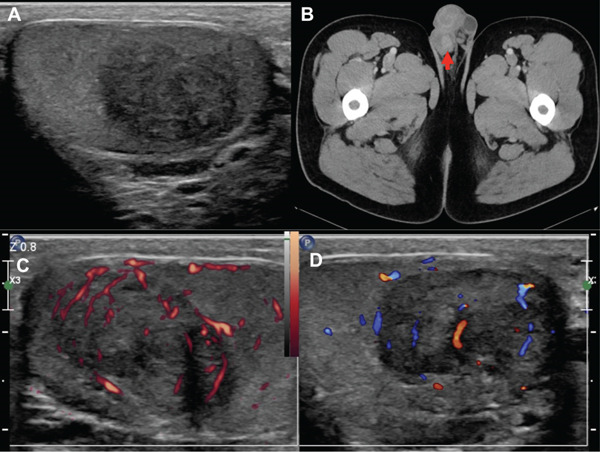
(A) Ultrasound of the right testis demonstrates a heterogeneous hypoechoic 1.3 × 2 × 2 cm lesion along the upper to interpolar region. (B) Non‐contrast CT showing a right testis mass red arrow. (C and D) Color Doppler.
